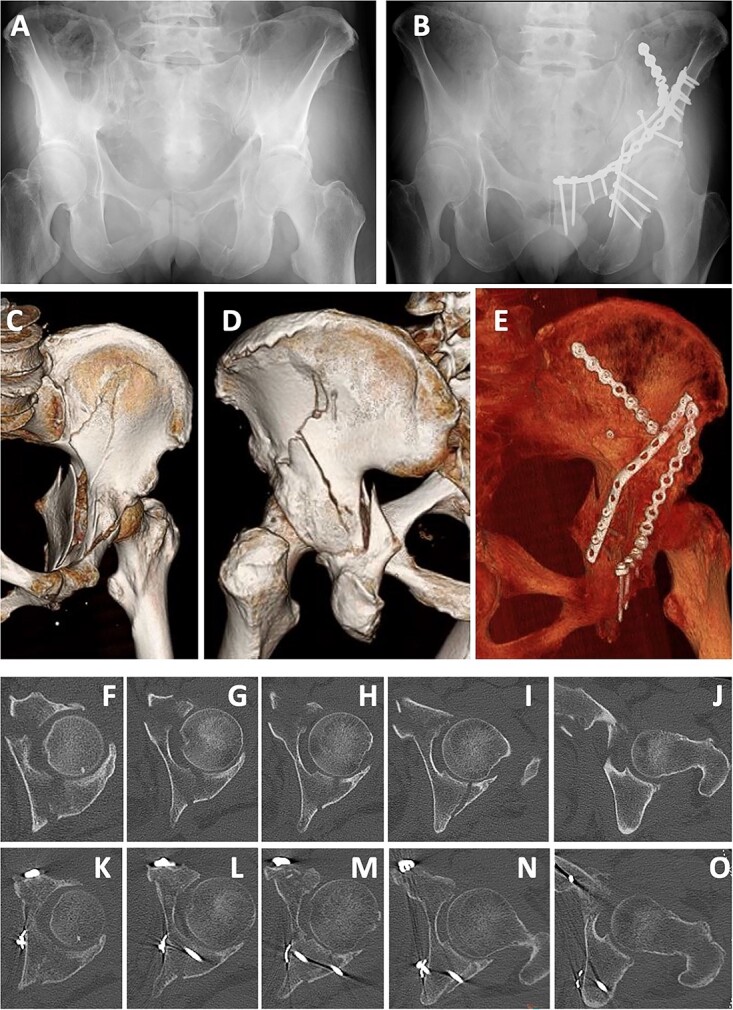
Case 1 images: (A) pre-operative X-ray, (B) post-operative X-ray, (C) pre-operative 3D-CT (anterior), (D) pre-operative 3D-CT (posterior), (E) post-operative 3D-CT, (F–J) pre-operative axial CT images, (K–O) post-operative axial CT.
